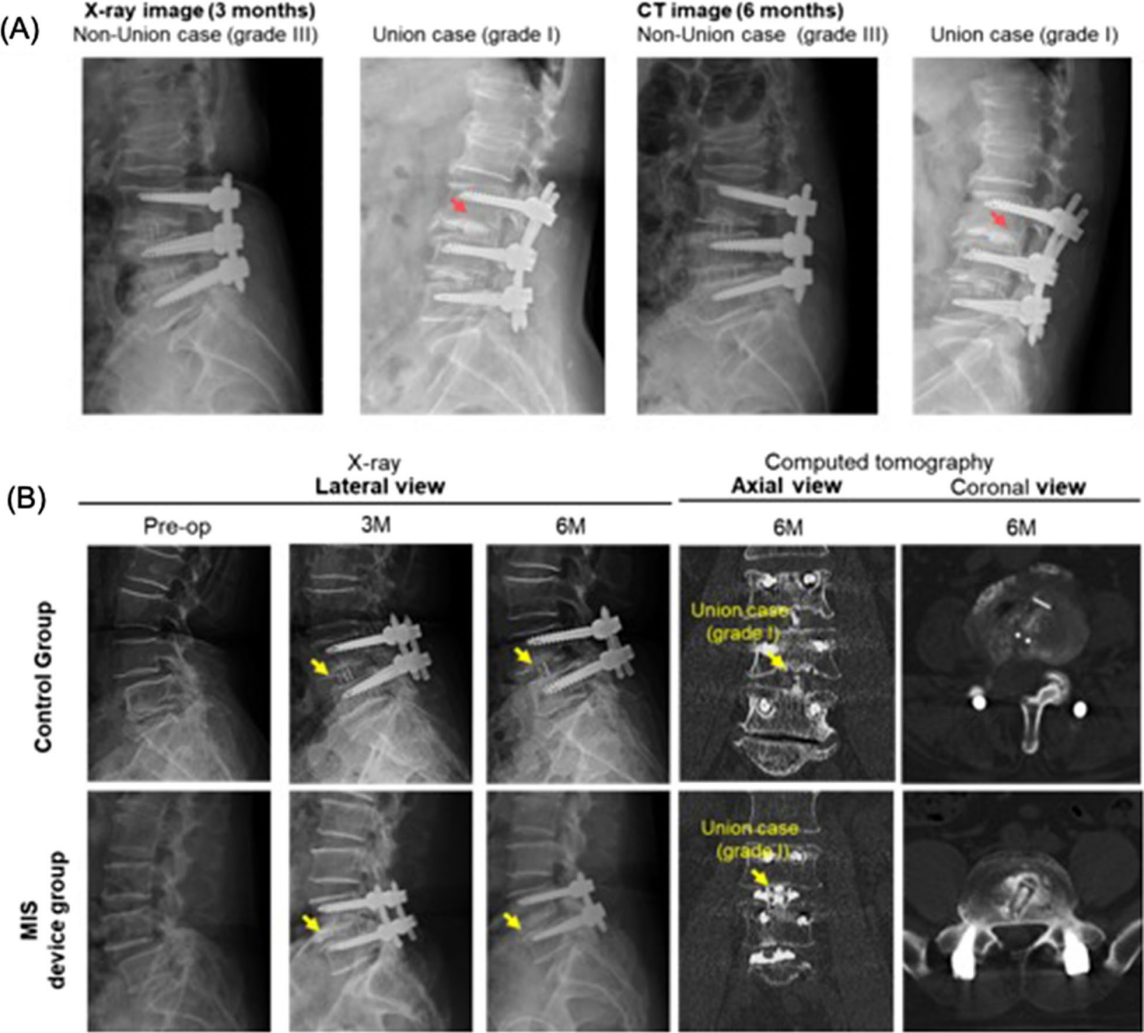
Representative radiography and computed tomography of surgical area of participant’s non-union and union cases. Representative radiography and computed tomography of surgical area of participant’s non-union and union cases. A X-ray image of the non-union and union case. Bone bridge (red arrow) was observed in the union case on X-ray taken 3 months post operation and the bone graft surrounding the cage (red arrow) was seen on X-ray taken 6 months after the surgery. B X-ray and CT images of the union cases receiving traditional or MIS graft delivery method. Bony formation and incorporation are indicated in yellow arrows
